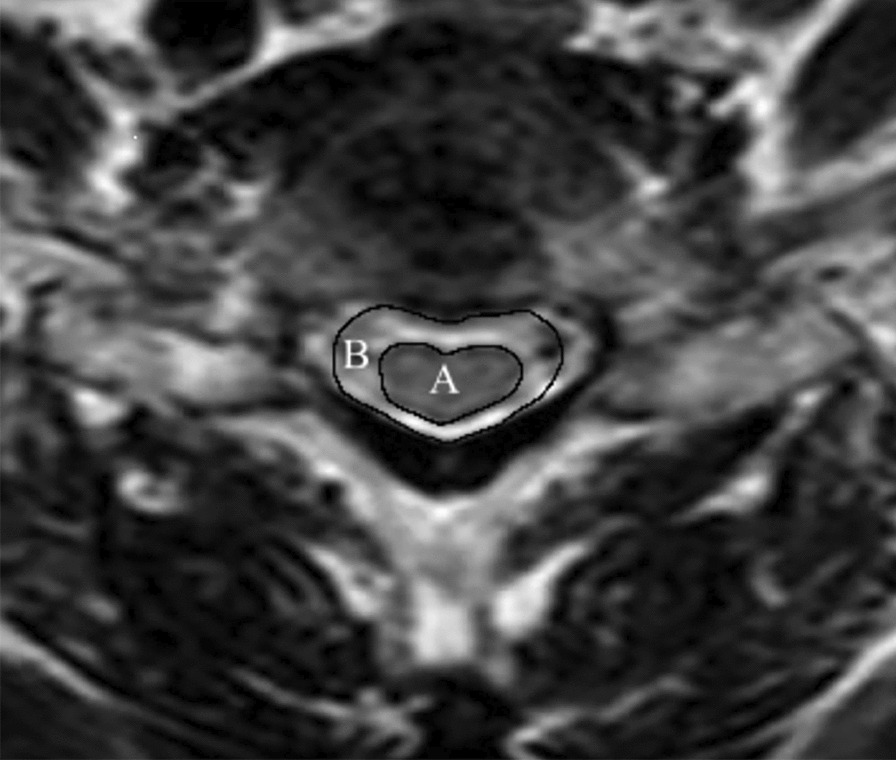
A The transverse area of the spinal cord and B the transverse area of the dural sac on T2-weighted axial MRI
